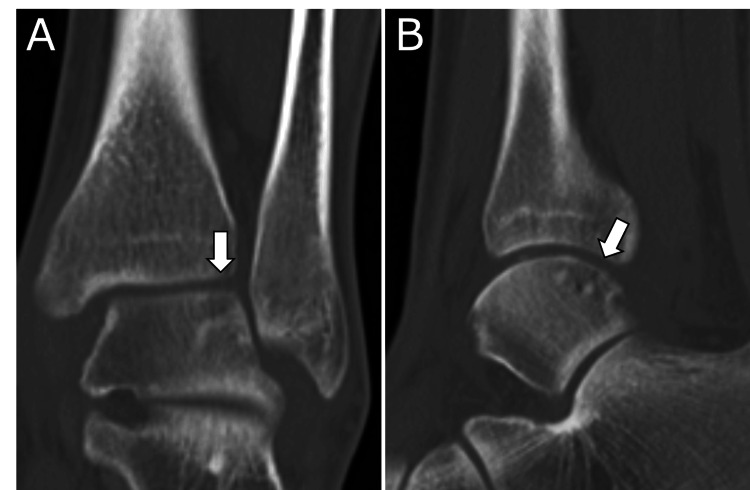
Plain computed tomography images at three months postoperatively Bone union of the osteochondral lesion is confirmed (arrow). A: Coronal view; B: Sagittal view
